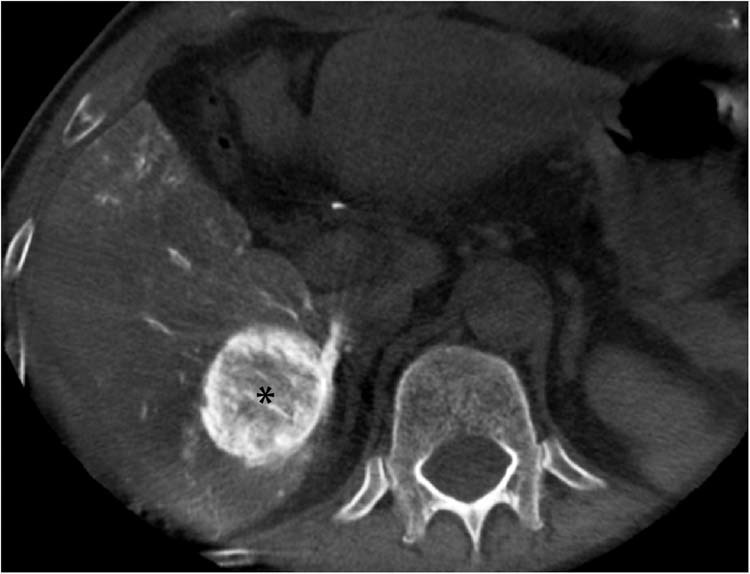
Contrast-enhanced cone-beam CT in the axial plane demonstrates homogeneous tumor enhancement and evenly distributed contrast medium within the tumor (asterisk).
